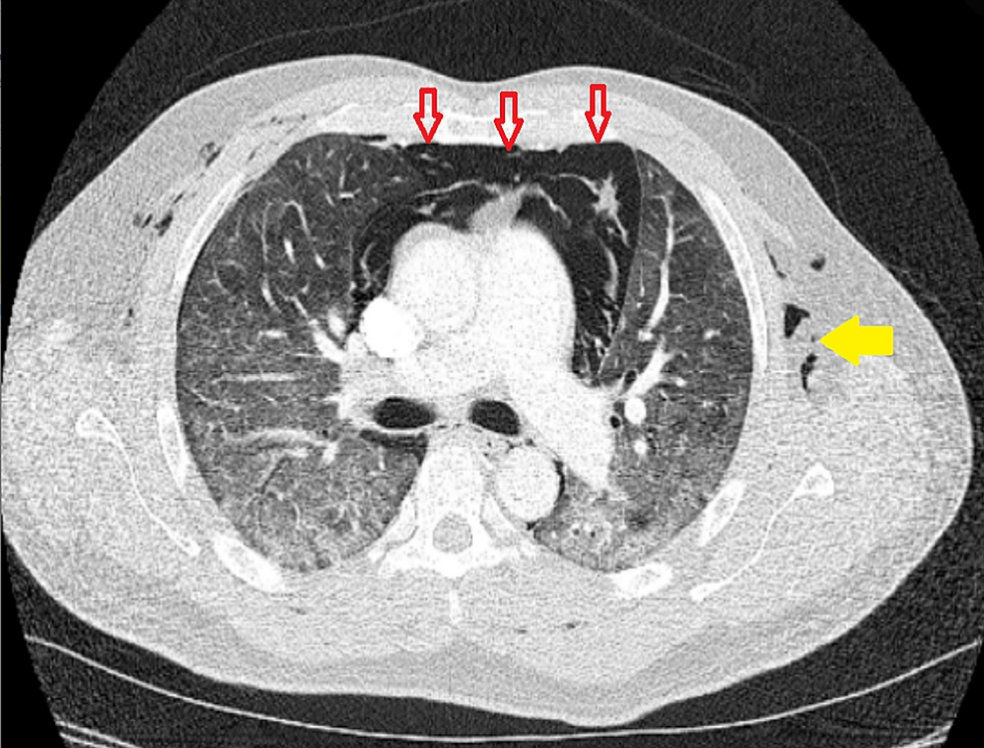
Chest CT axial section of pulmonary parenchymal window showing extensive ground glass opacities in both lung fields, along with subcutaneous emphysema (yellow arrow), and pneumomediastinum (red arrows).
